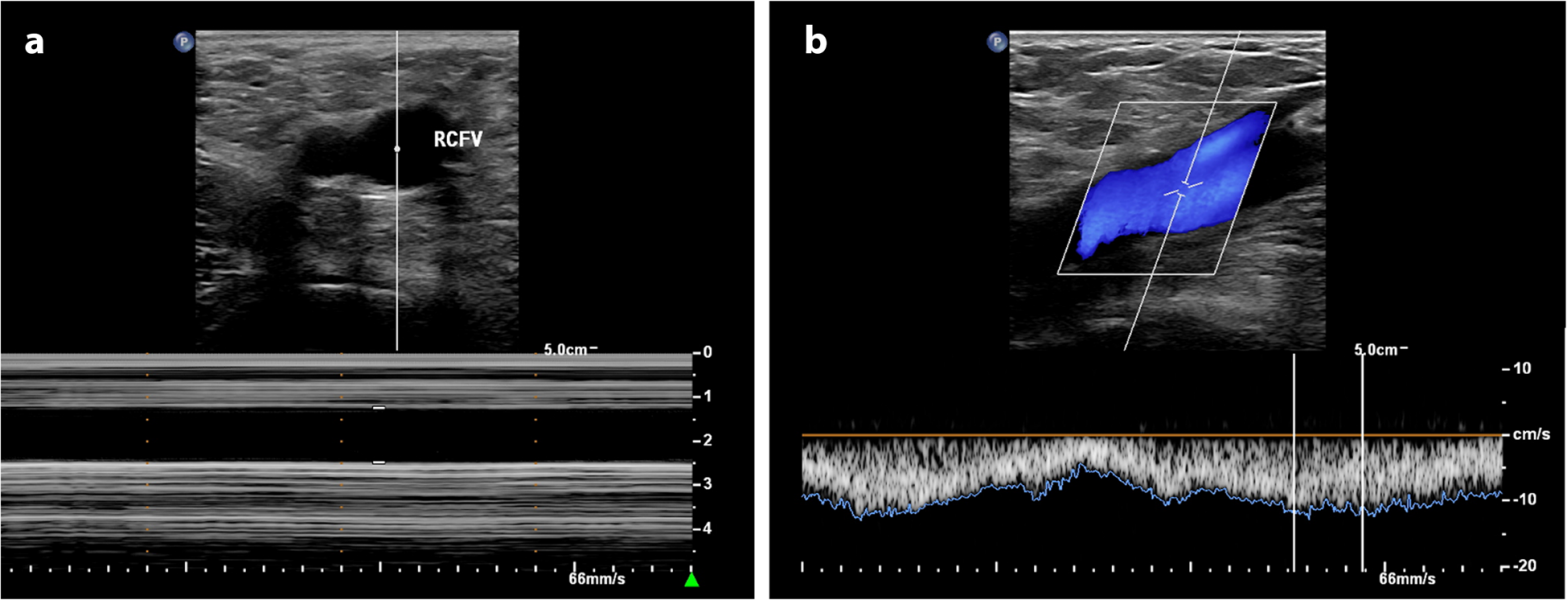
Measurement of RCFV by ultrasound with the high frequency linear array probe. a M-mode image showing the transverse diameter of RCFV; b Pulsed-wave Doppler-mode image showing the peak velocity of RCFV. RCFV, right common femoral vein
